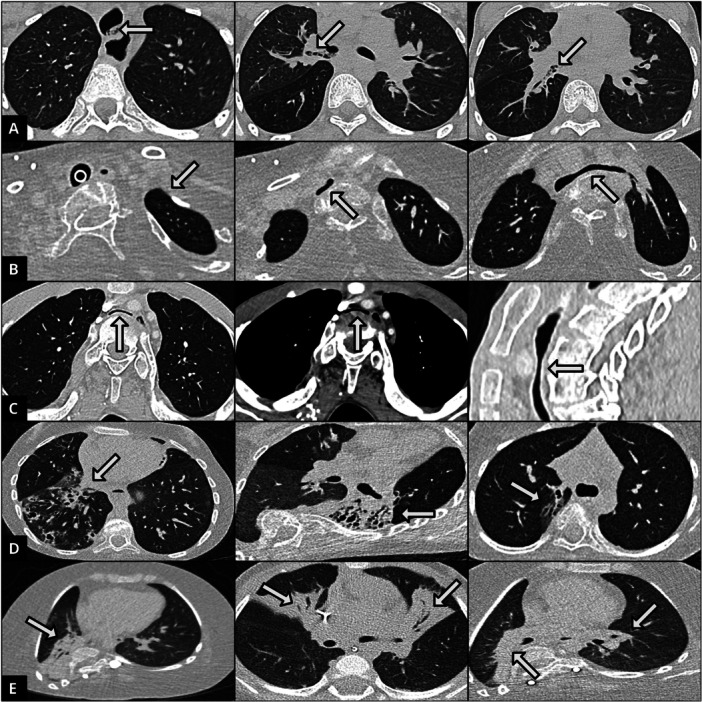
Representative chest computed tomography (CT) findings in children with severe neurological disorders. (A–C) CT scans from a single patient demonstrating retained secretions within the central tracheobronchial tree (A) and subsequent tracheal stenosis secondary to severe thoracic deformity (B, C). (D) Bronchiectasis in a second patient. (E) Lung consolidations in a third patient. Pathological findings are indicated by arrows.
